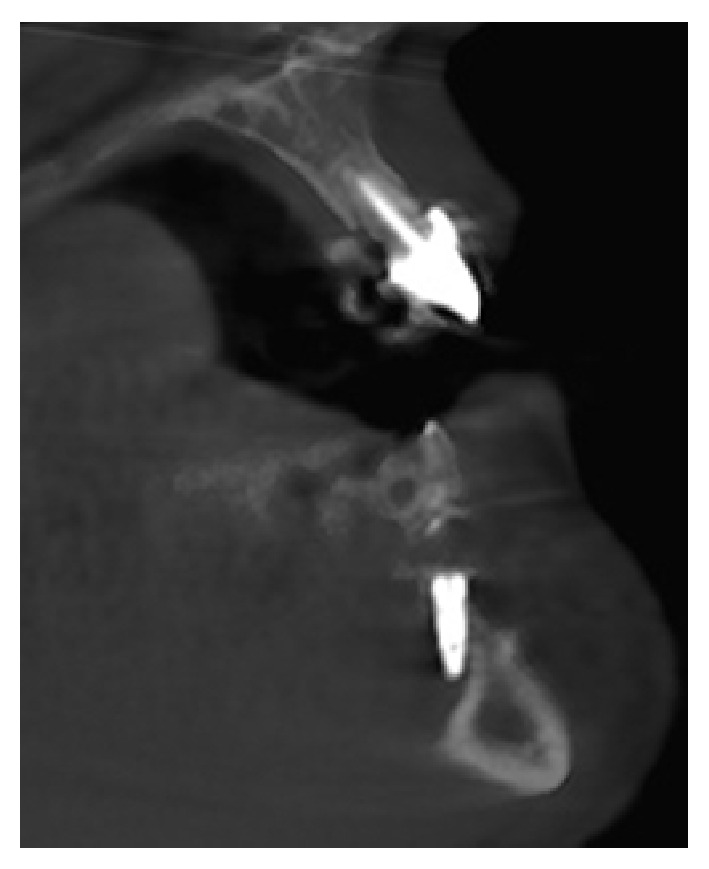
Cone beam tomography of the implant in region 32.
